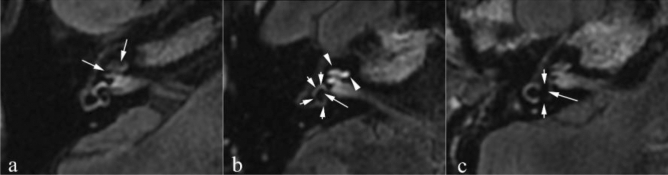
Cochlear and vestibular endolymphatic hydrops with different degrees were shown. High-resolution 3D-FLAIR images were obtained 6 h after intravenous gadolinium injection for patients with Ménière’s disease. A 45-year-old man with right Ménière’s disease (a): Axial 3D-FLAIR image showed that the scala vestibuli was fully obliterated due to the dilated cochlear duct (arrow) (cochlear hydrops grade II). A 49-year-old man with right Ménière’s disease (b): Axial 3D-FLAIR image showed a confluence of the saccule and utricle (long arrow) with a continuous peripheral rim enhancement of the perilymphatic space (short arrows) (vestibular hydrops grade II). Note: abnormal cochlear perilymphatic enhancement (arrowhead) was shown on the same side. A 48-year-old woman with right Ménière’s disease (c): Axial 3D-FLAIR image showed that the perilymphatic enhancement was no longer continuous. There was a full obliteration of the bony vestibule, and the perilymphatic enhancement was no longer visible (short arrow) (vestibular hydrops grade III).
